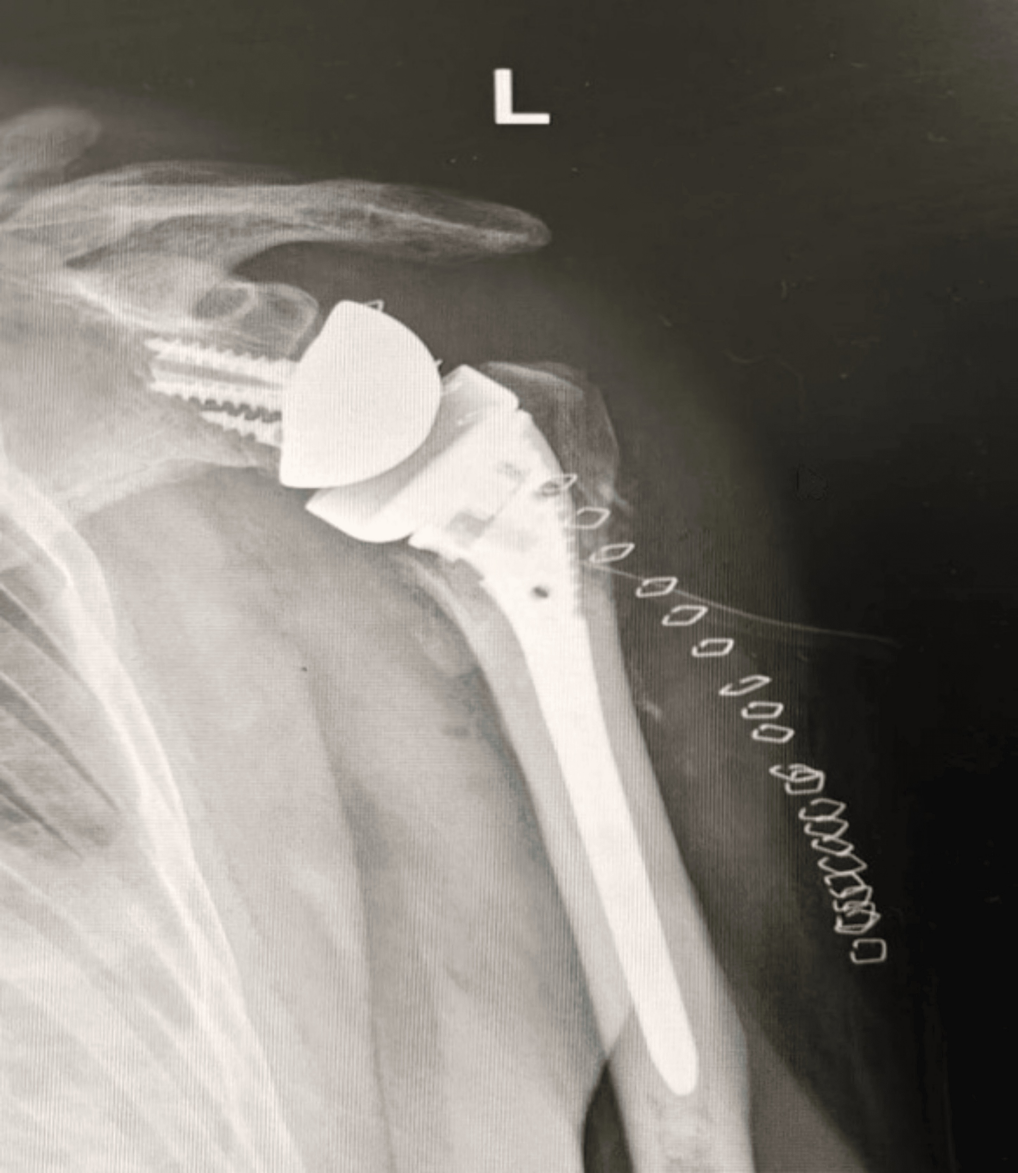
Post operative anteroposterior radiograph after reverse shoulder arthroplasty (RSA) Image Credits: First Author
